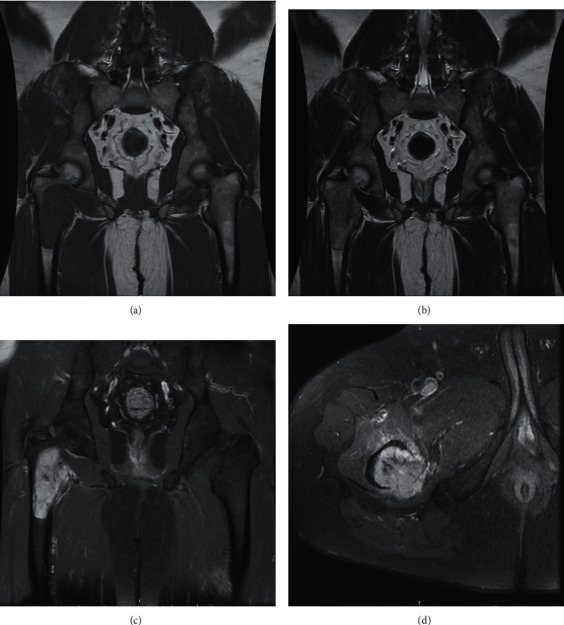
The MRI revealed the tumor at right proximal femur with soft tissue extension, especially at posteromedial aspect: MRI coronal T1 weight (a), coronal T2 weight (b), coronal T1 + contrast (c), and axial T1 + contrast (d).
